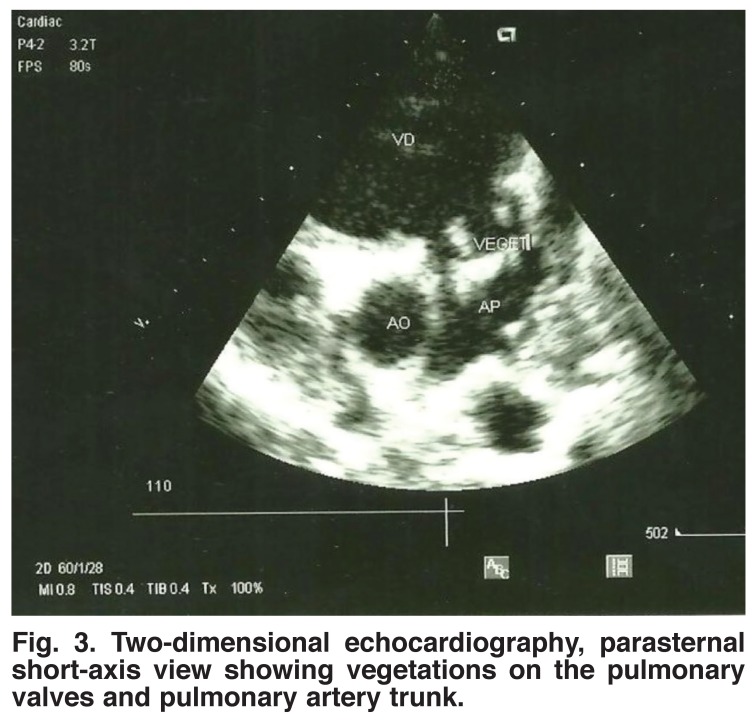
Two-dimensional echocardiography, parasternal short-axis view showing vegetations on the pulmonary valves and pulmonary artery trunk.
